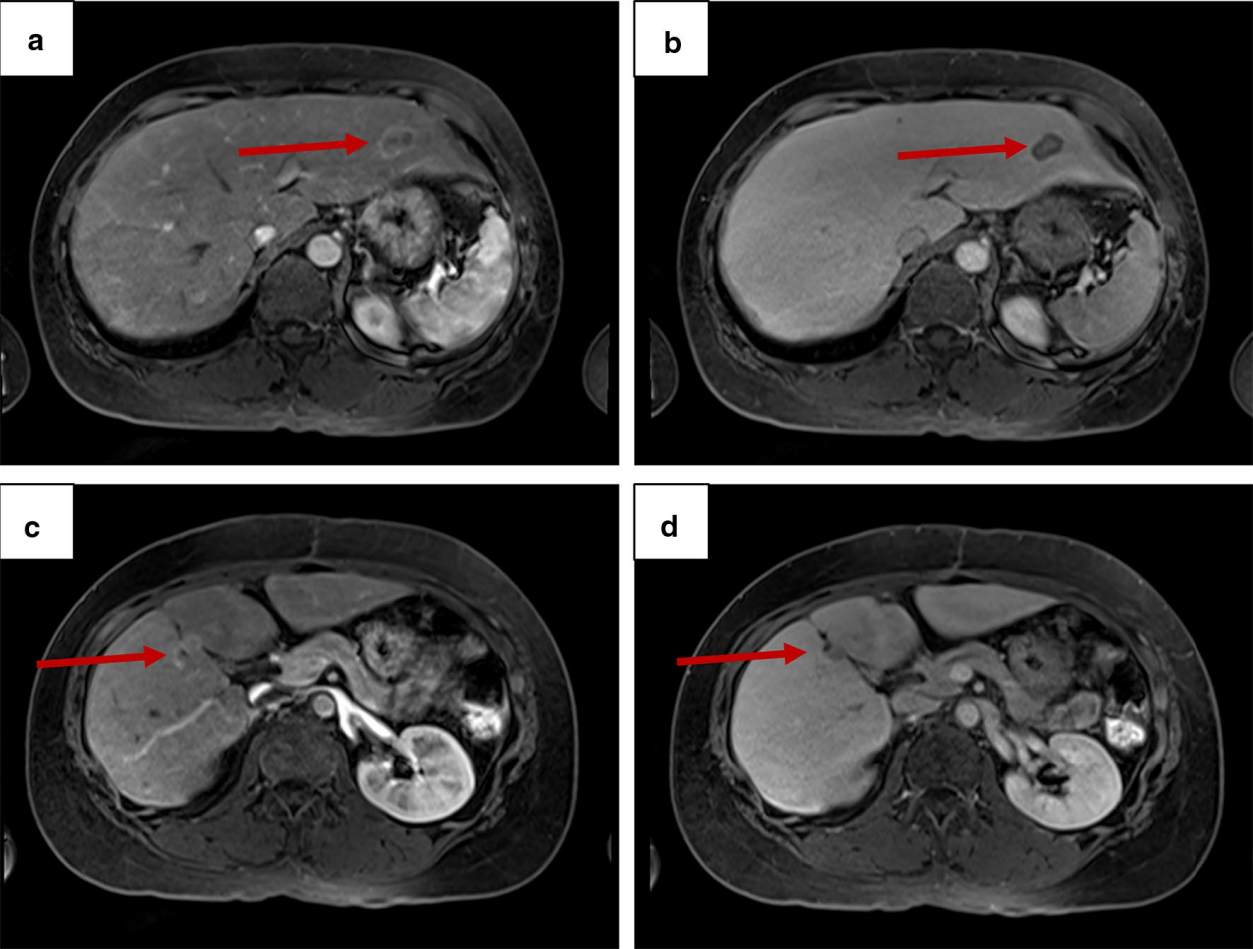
Recurrence in the liver 1 year after the hepatectomy. Gadoxetic acid-enhanced magnetic resonance imaging showing two liver lesions in the segment 2 (a and b) and segment 4/5 (c and d) with hyperenhancement on arterial phase (a and c) and defect on hepatocyte phase (b and d). Arrows indicate the lesions
